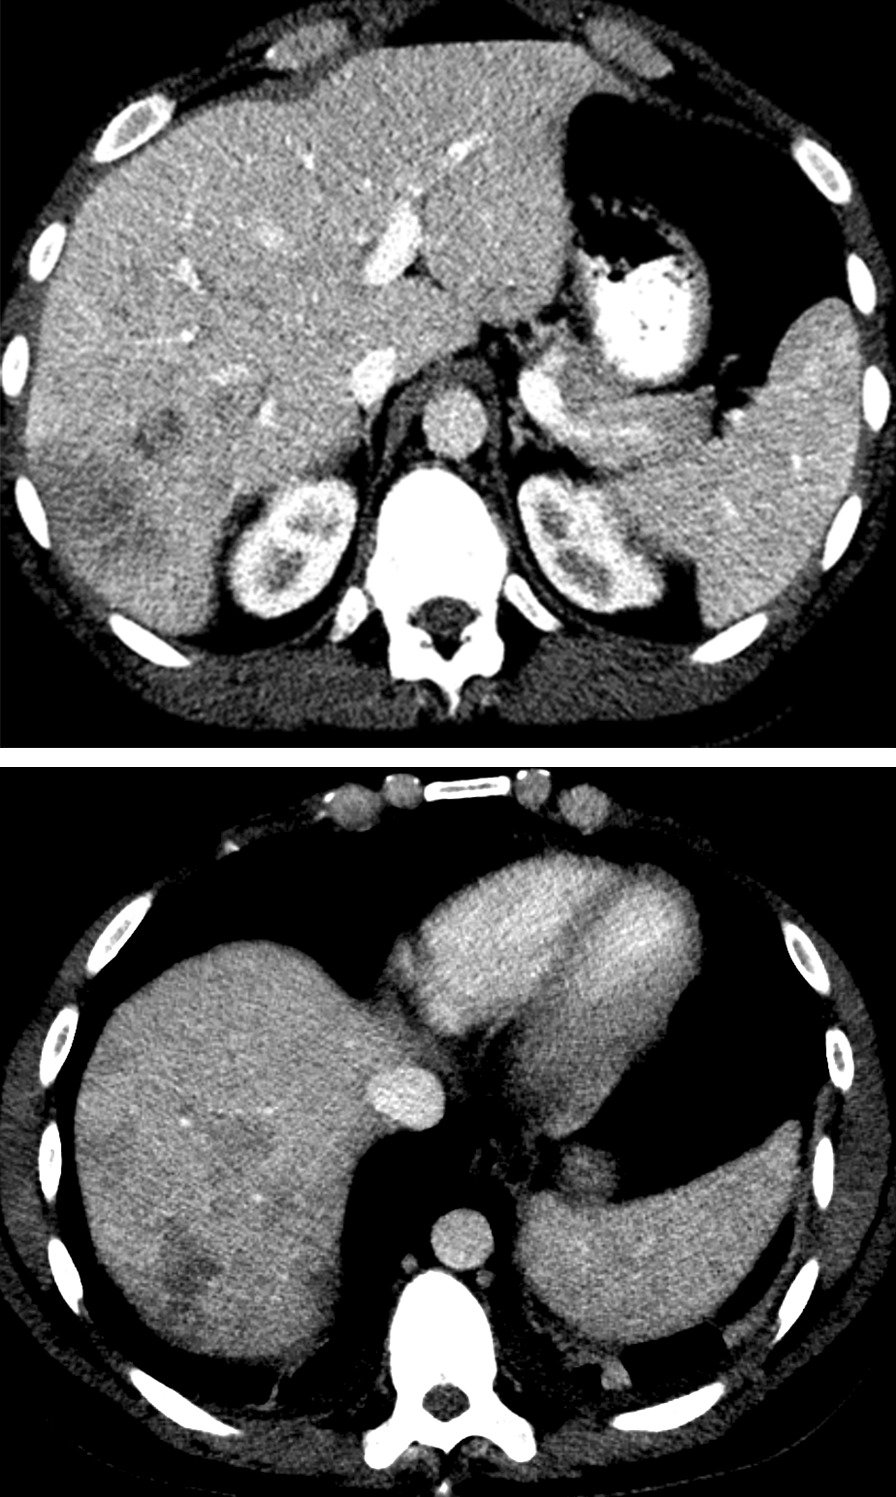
Computed tomography with oral and intravenous contrast agent, showing irregular hypodense liver lesions in segment VI and VII in portal-phase contrast-enhanced CT, corresponding to the ultrasound findings
